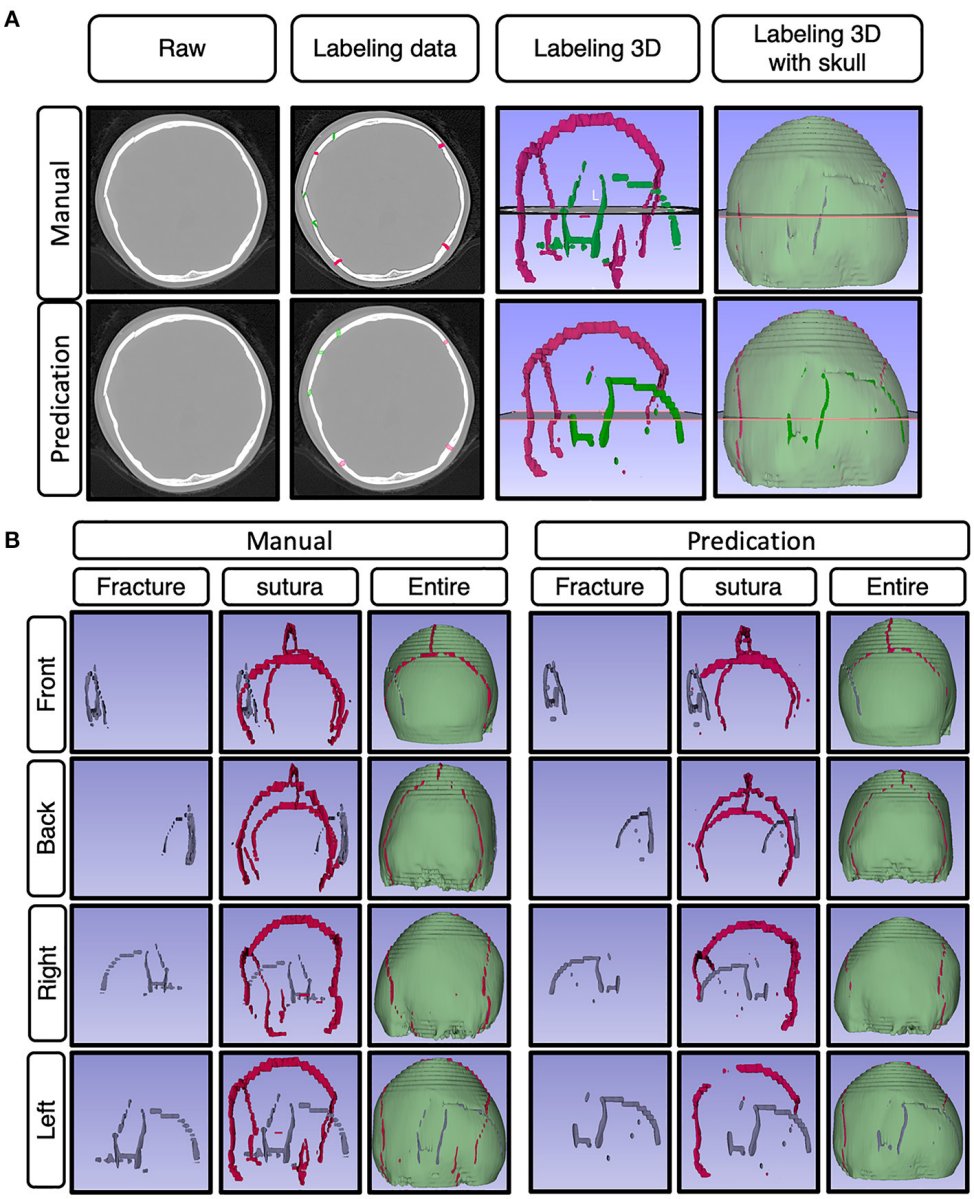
Represented images for the demo case. (A) Representative images showing the raw data of skull fractures based on CT bone algorithm sequence slices, manual skull fracture labeling, 3-D reconstruction images of sutura and fracture, and 3-D reconstruction images of sutura, fracture, and whole skull. (B) The represented images show the demo case from a 3D perspective (front, back, right side, left side), with manually labeled images and predication-labeled images.
